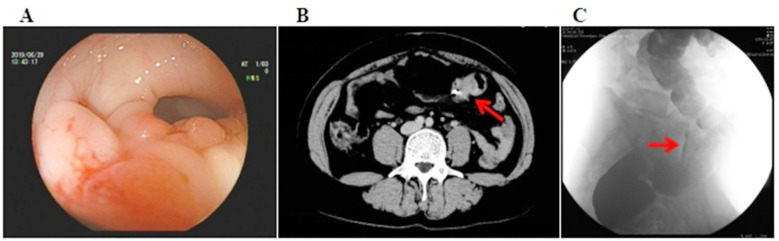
A, Colonoscopy showed an irregularly protrude lesion in the sigmoid colon about 19cm from the anal verge. The surface was rough and granular, invading about 1/2 of the bowel, and the bowel lumen was stiff with stenosis. B, Pelvic CT scan showed local thickening of the intestinal wall in the left lower abdomen, and the metal artifact was a titanium clip (which was clipped during the colonoscopy and can be x-rayed before surgery to determine the location of the mass). C, Barium enema showed mucosal abnormality and focal stenosis of the sigmoid region. The rest of the colon was normal.
